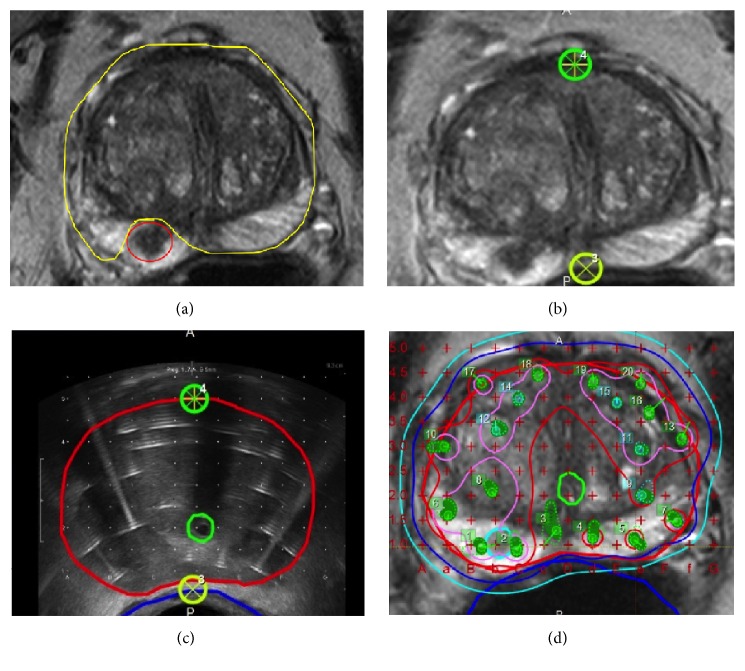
(a) Preprocedural MRI demonstrating GTV (yellow) and nondiseased prostate (red). Preprocedural MRI using two anchor points (green and yellow circles) (b) are able to be registered to intraprocedural TRUS with 2.5 mm grid spacing (c). (d) The resulting merge of the TRUS grid and preprocedural MRI with GTV (thick light blue), urethra (thick solid light green), rectum (thick blue line), and isodose lines (thin lines) for typical whole gland plan using 18 HDR catheters.
